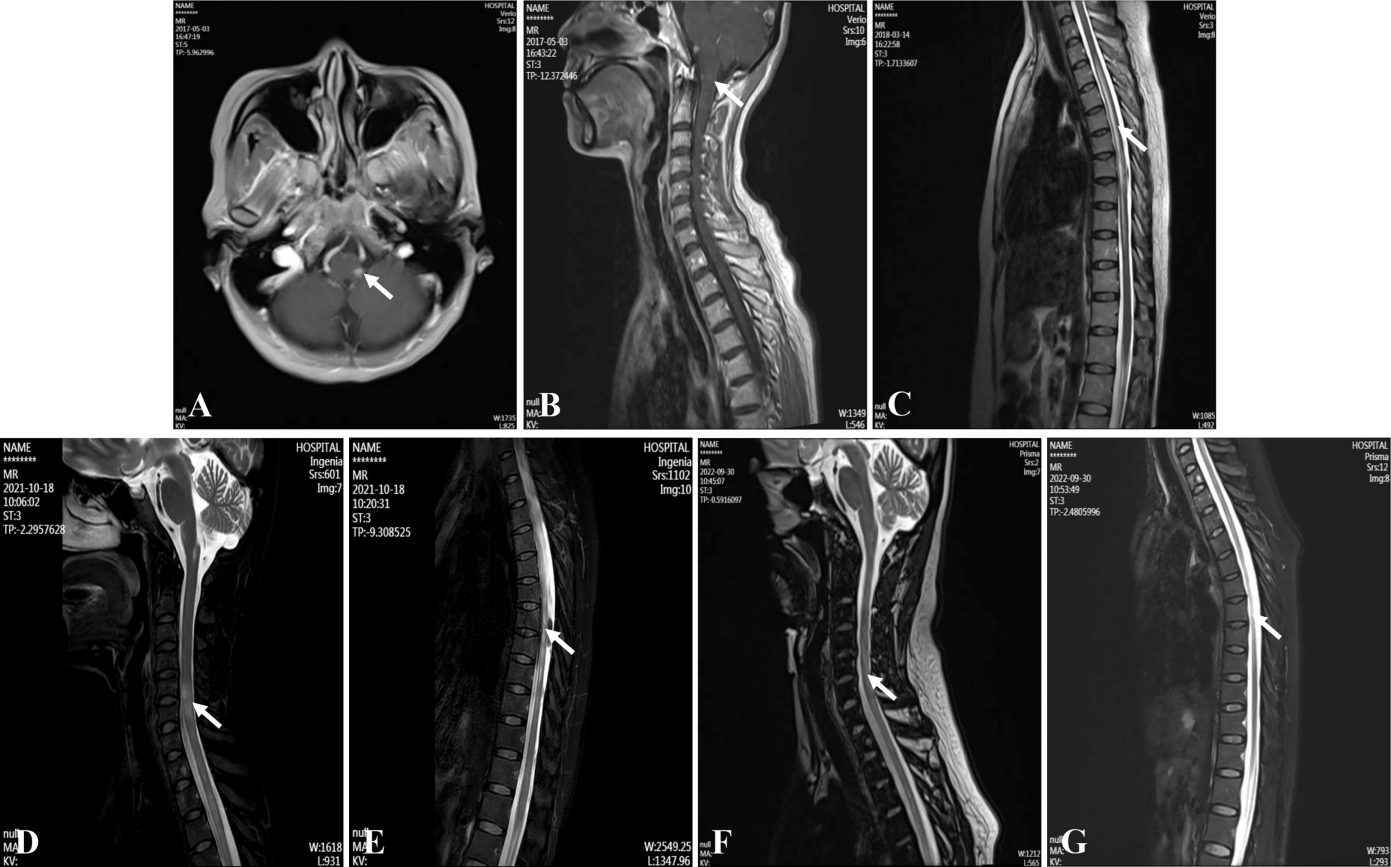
Magnetic resonance imagings of the brain and spinal cord. (A, B) Medulla oblongata lesions at the first attack. (C) Thoracic cord lesions at the second attack. (D, E) Cervical and thoracic cord lesions at the last attack. (F, G) Cervical and thoracic cord lesions at four months after using ofatumumab.
